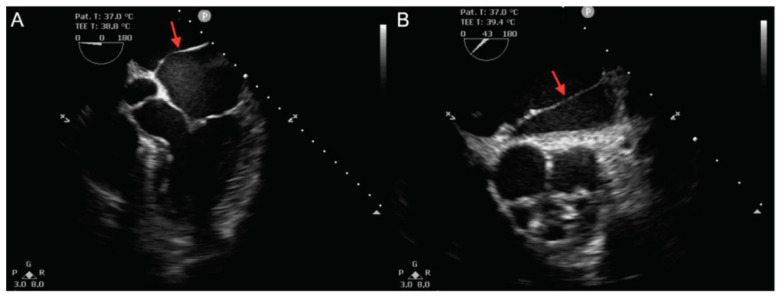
Transesophageal echocardiography. (A): Mid-esophageal 5-chamber view and (B): Mid-esophageal short-axis view, showing a membrane (red arrows) separating the left atrium in two separate chambers.
